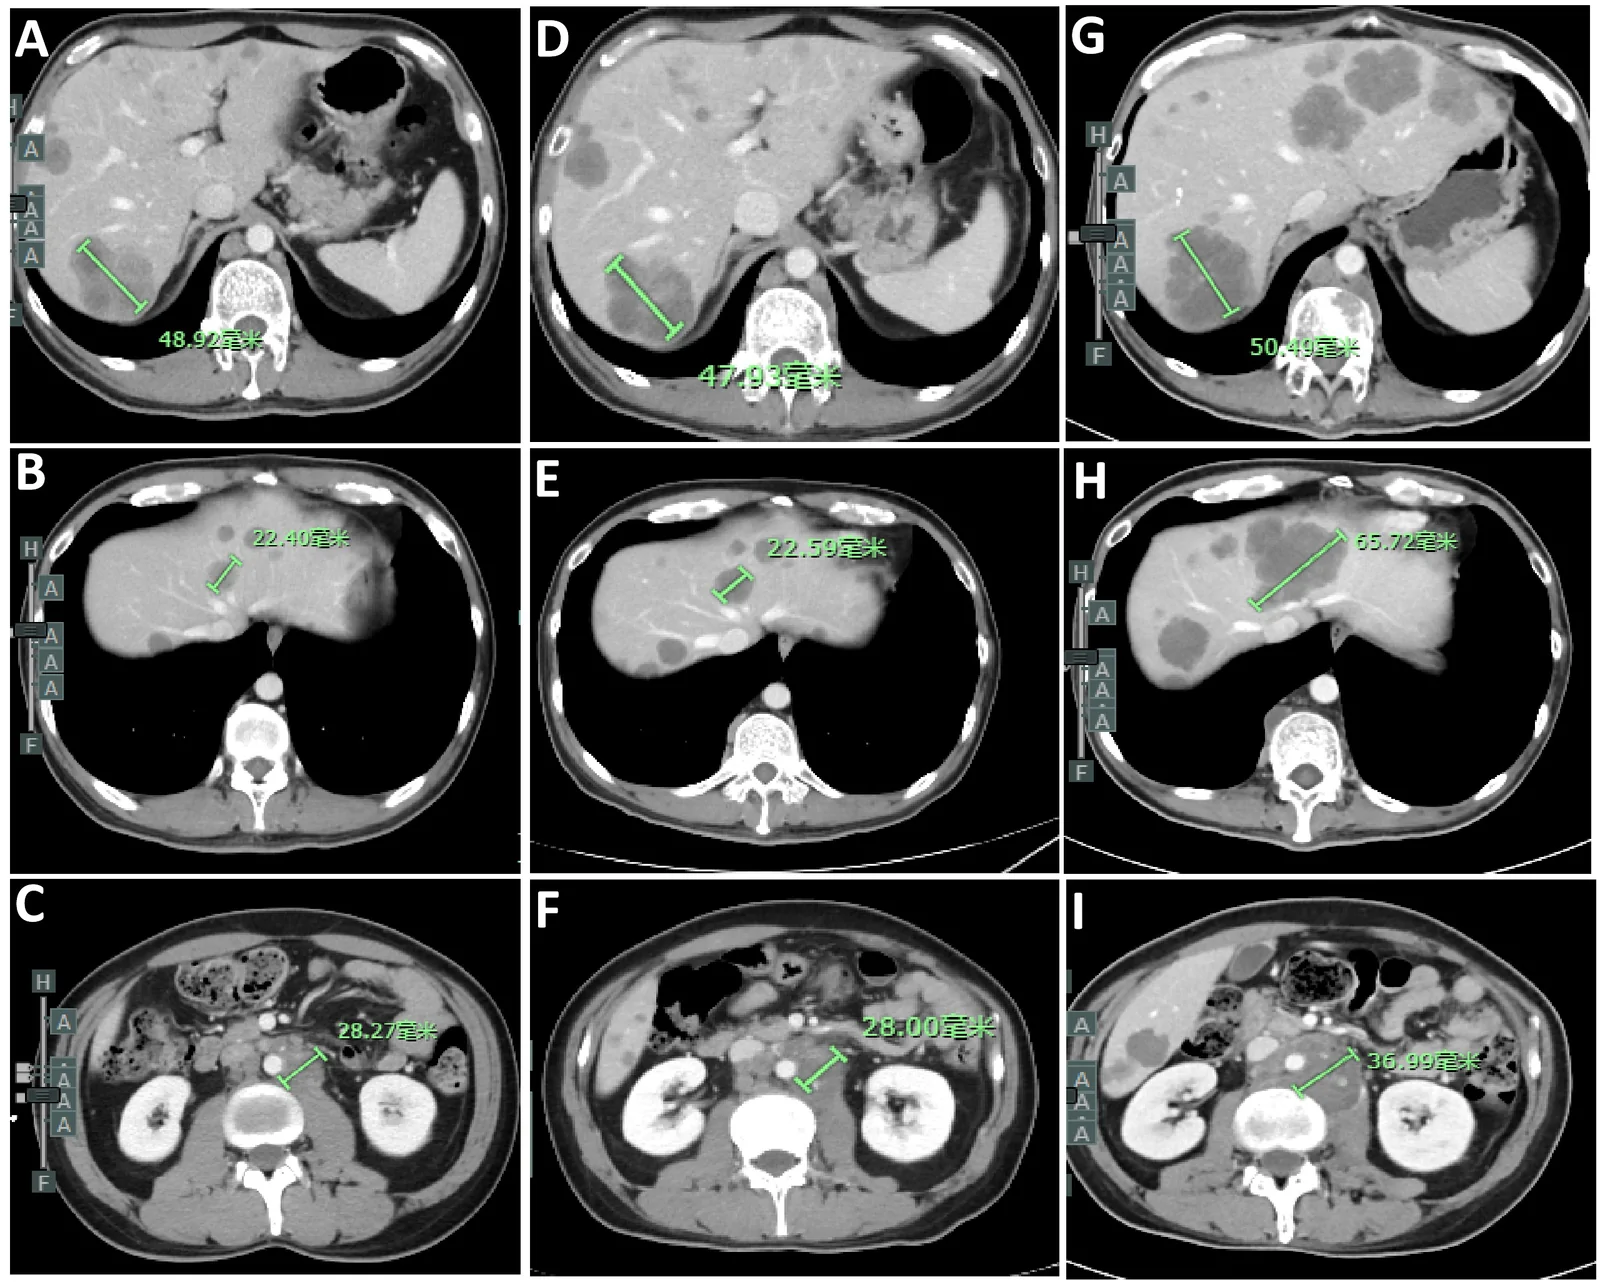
Serial CT images during seventh-line bevacizumab plus sintilimab and chidamide therapy. (A–C) Baseline (April 1, 2024). Sum of target lesions: 9.9 cm. (D–F) Best response (May 16, 2024). Sum of target lesions reduced to 9.8 cm (−1.0%); per RECIST, best overall response: stable disease (SD). (G–I) Progression (September 24, 2024). Sum of target lesions enlarged to 15.0 cm (+51.5% vs. baseline); per RECIST, progressive disease (PD). Scan dates are shown in each panel. All images are at comparable anatomical levels.
